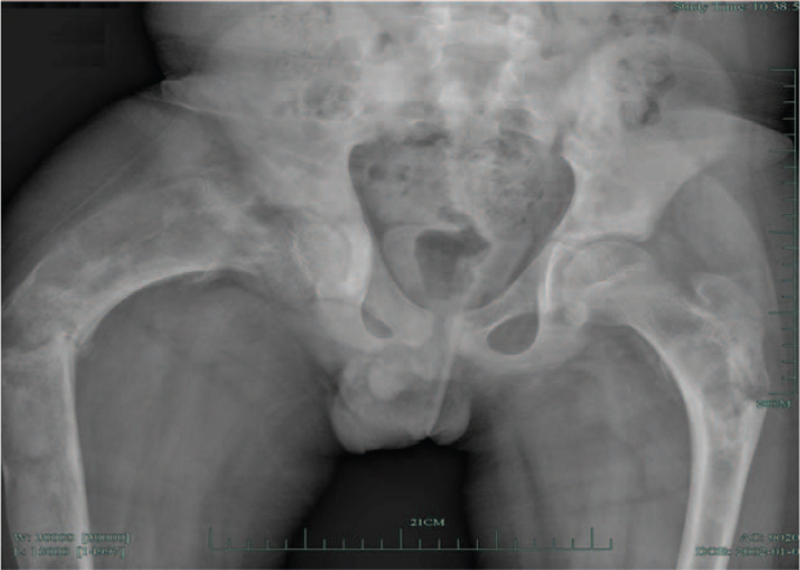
X-rays of both the femor showed extensive bowing deformities and right femur fracture.
